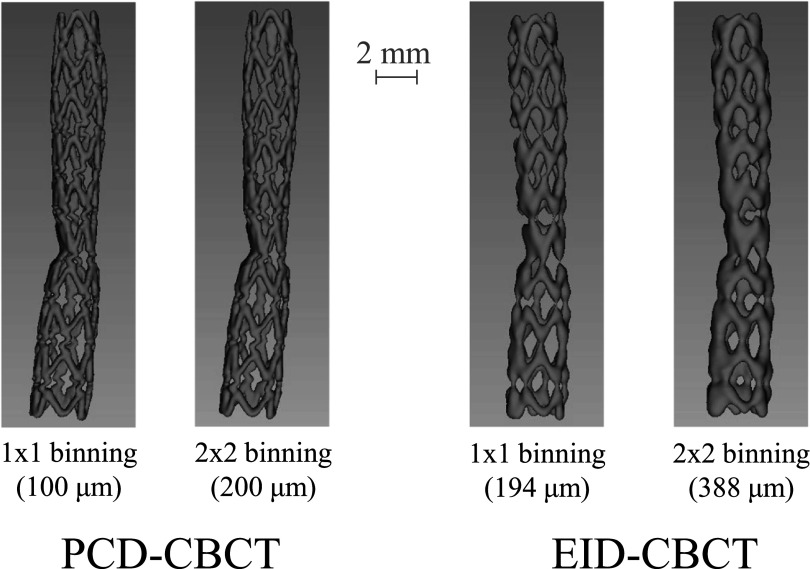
UHR PCD-CBCT and EID-CBCT 3D reformatted images of the 3 mm stent under different detector pixel binning conditions. The PCD-CBCT demonstrates improved delineation of the stent wires even when the PCD pixels are binned to match the pitch of the unbinned EID pixels. The pure EID images are displayed to compare to the PCD image rather than displaying a fused PCD-EID image.
